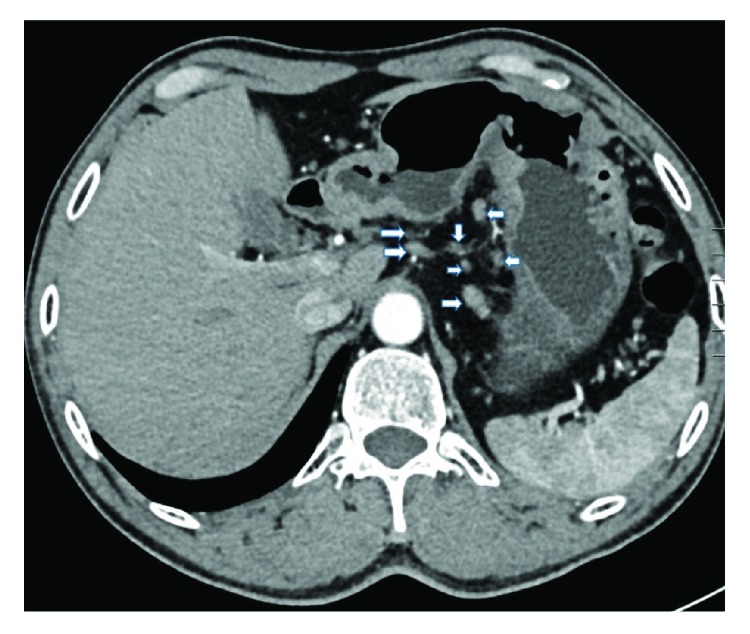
CT image obtained in a 50-year-old man. Isolated but multiple (>7) lymph nodes are seen around the lesser curvature of the stomach.
